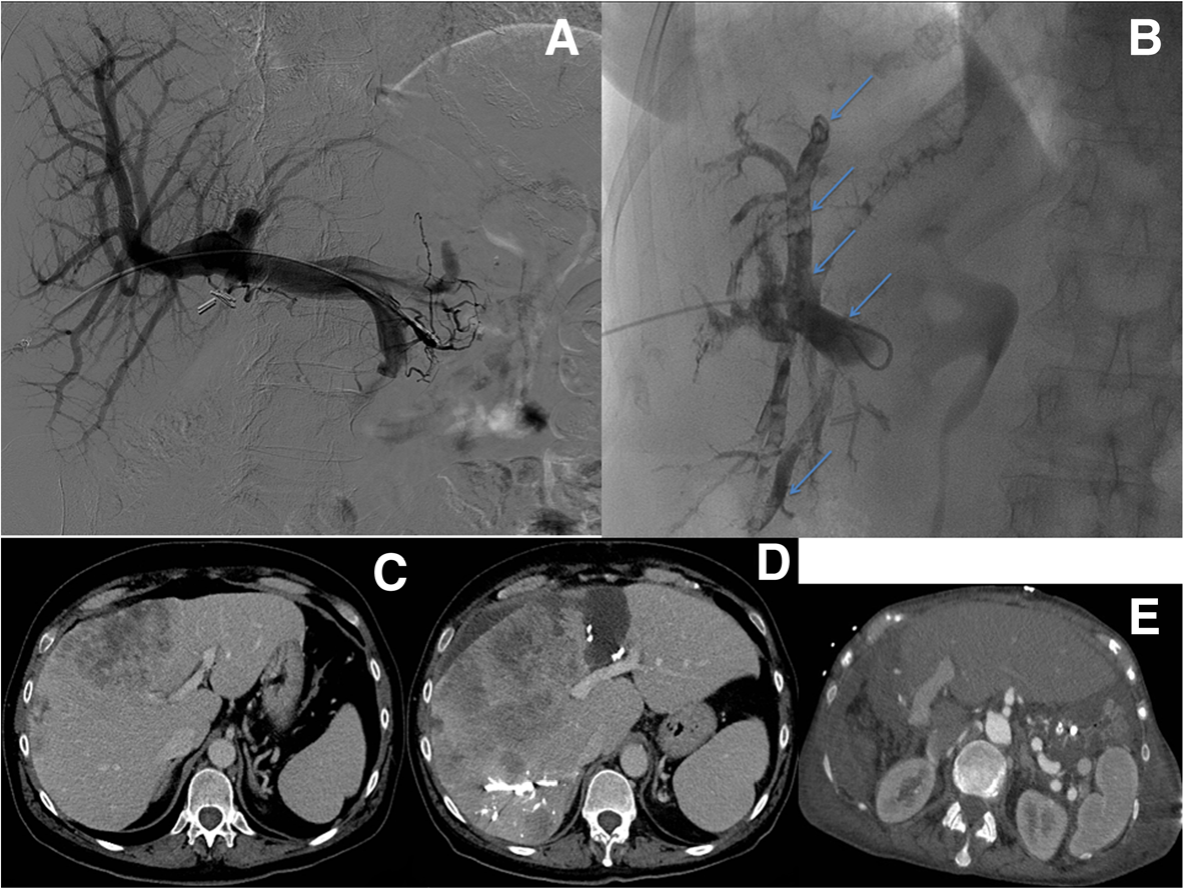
Portal Vein Embolization (PVE) before right hepatectomy in a patient affected by Cholangiocarcinoma. a Preliminary portography before right PVE. b Right PVE using PVA particles and Squid-12 (light blue arrows). c Contrast enhanced CT pre-PVE showing an insufficient volume of left hepatic lobe. d Contrast enhanced CT post-PVE showing an increase of left hepatic lobe, with an increase of FLR-V of 71%. e Contrast enhanced CT post- right hepatectomy shows hypertrophy of the left hepatic lobe (FLR)
